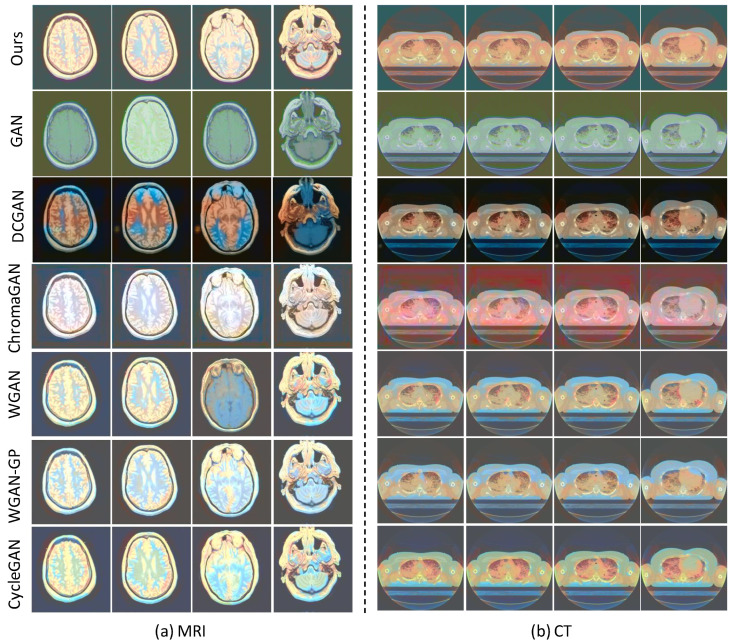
Qualitative colorization results of MRI images and CT images of the VHP dataset using different GAN-based image colorization methods.
